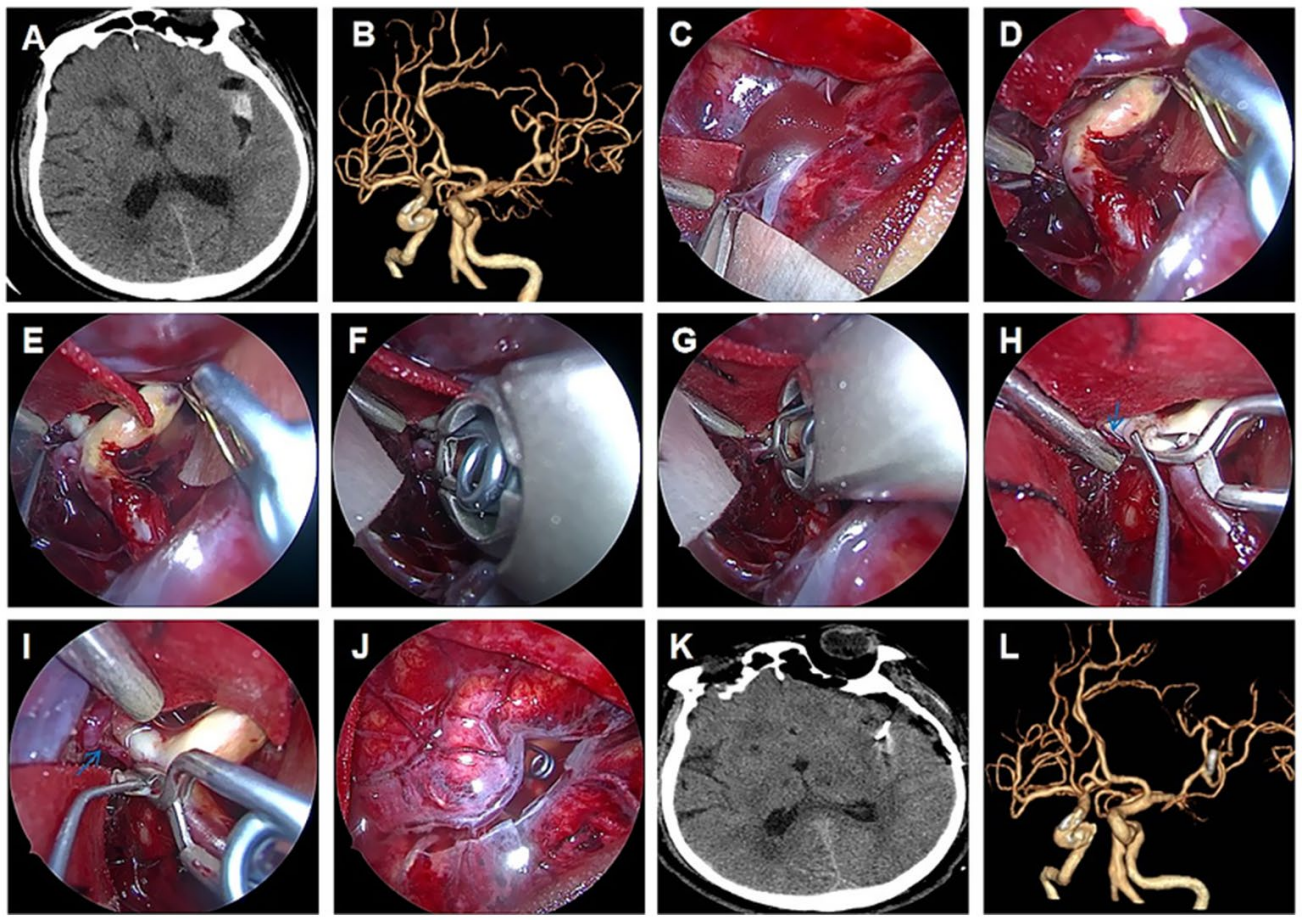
Endoscopic clipping of MCA aneurysm demonstrating retractorless exposure. Emergent CT demonstrated SAH (A) and a subsequent MCA aneurysm on CTA (B). Fully endoscopic opening of the Sylvian fissure (C). Fully endoscopic exposure of the ruptured aneurysm (D,E). Fully endoscopic dissection and clipping of an aneurysm (F,G). Post-clipping inspection indicated complete occlusion of the aneurysm and preservation of the branch artery and perforators (arrow) (H,I). No injury of the blind area posterior to the head of the lens (J). None of the postclipping cerebral infarctions occurred on CT (K), and CTA before dismission confirmed complete occlusion of the aneurysm (L).
